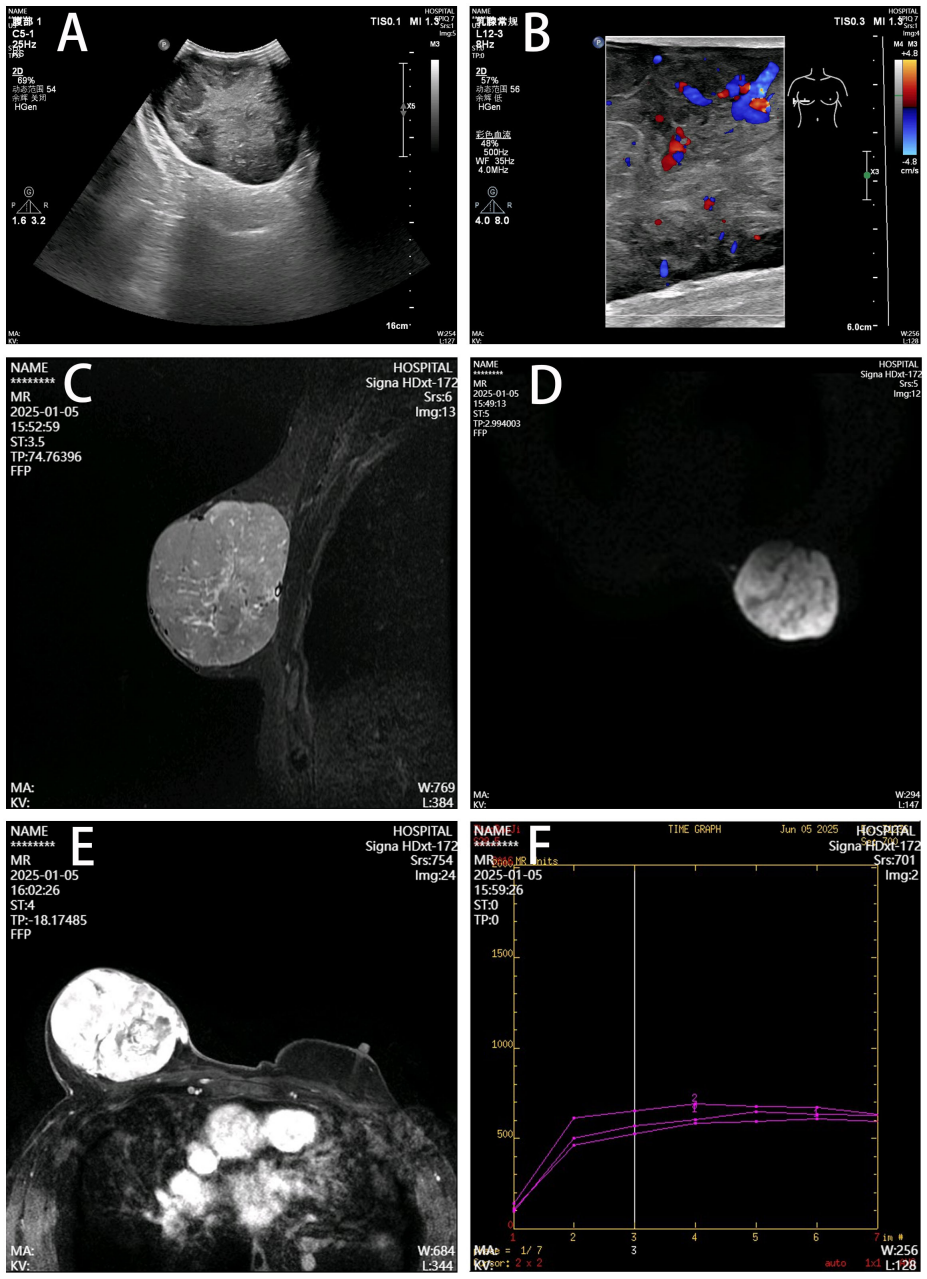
Imaging features of the right breast mass. (A) Ultrasonography, Well-defined hypoechoic mass (12.3×9.4×5.5 cm) with heterogeneous echogenicity. (B) Color Doppler, Moderately abundant internal blood flow. (C) T2 fat-suppressed MRI, Lobulated mass showing heterogeneous hyperintensity (arrow). (D) DWI, Hyperintense signal with clear margins. (E) Contrast-enhanced MRI, Marked heterogeneous enhancement with capsular discontinuity (arrowhead). (F) Time-intensity curve, Rapid initial rise followed by plateau kinetics.
